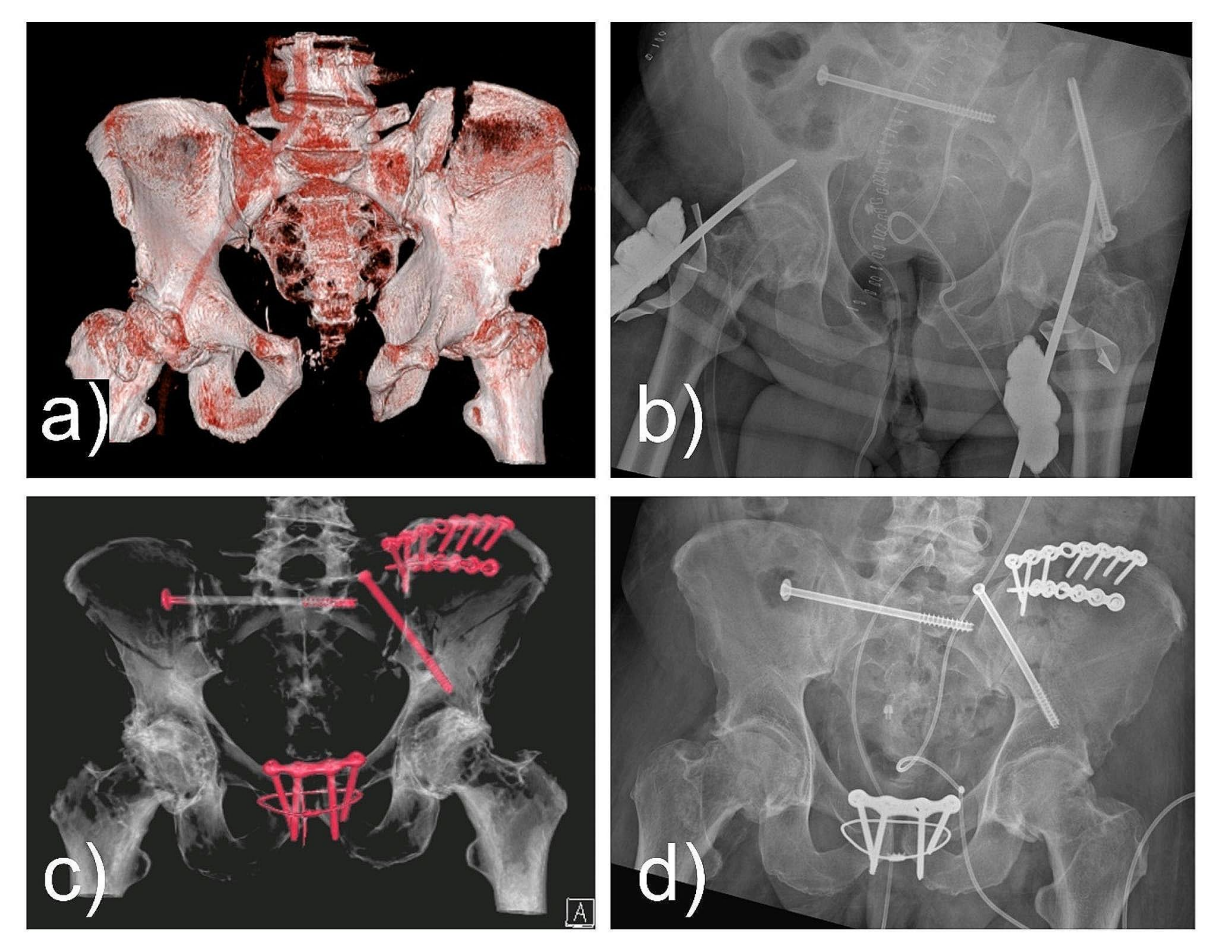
Case#3: a) CT scan of a pelvic ring injury with widening of the pubic symphysis, the iliosacral joint and fracture of the ilium (AO: 61C2.1d). Further the patient had a severe kidney laceration with significant hemorrhage and destruction of the ureter. b) Emergency treatment was done with external fixation and SI- and supraacetabular screw placement. c) Final treatment was done using symphyseal plating with TOCF, plating of the ilium and screw-revision to a LC-screw. d) The pelvic ring injury healed but the patient had a prolonged in-hospital stay because of the kidney injury
